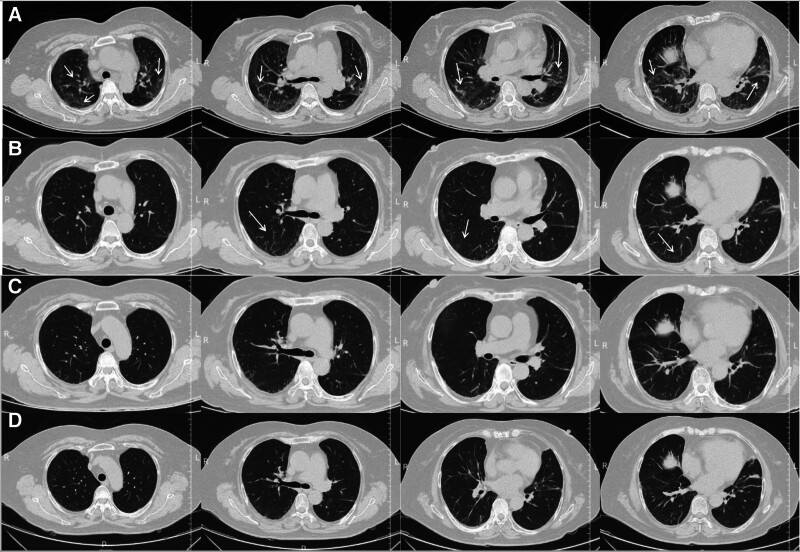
Chest CT changes. (A) Upon admission, chest CT showed interstitial change where linear and patchy high-density shadows were seen subpleurally, bilateral pleural hypertrophy and mild pleural effusion. (Arrows). (B) Two weeks after anti-infection therapy, linear and patchy shadows reduced. (Arrows). (C) One month after admission. (D) Last CT reexamination of 1st hospitalization. CT = Computed tomography.
